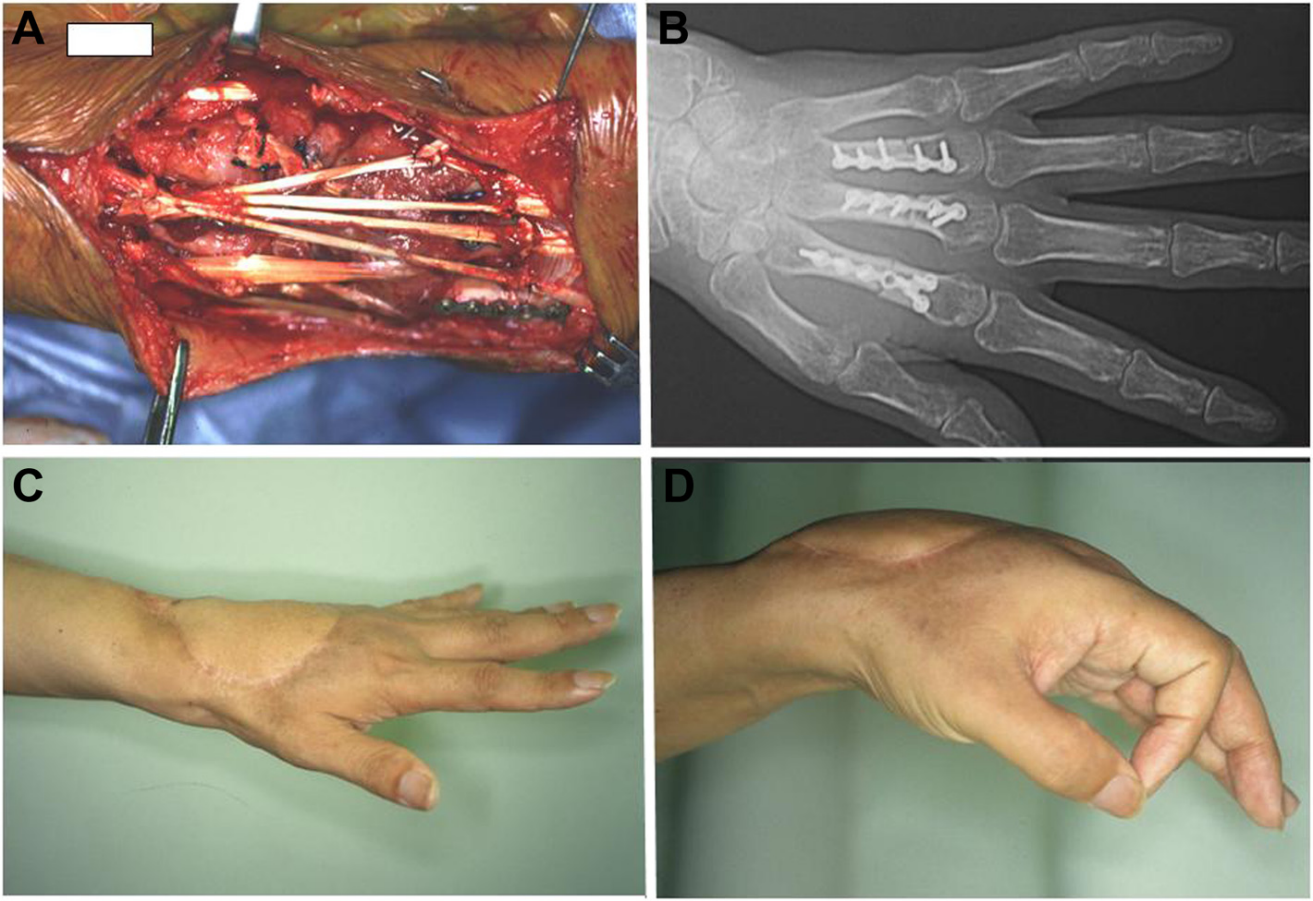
Intraoperative view and postoperative hand function. (A) The extensor digitorum muscles and bones were re-implanted to the original corresponding structures. (B) Postoperative radiograph. The metacarpal bones were fixed with the phalangeal plates. (C, D) The patient could extend his fingers and wrist due to the re-sutured tendons. MSTS rating system was 24.
